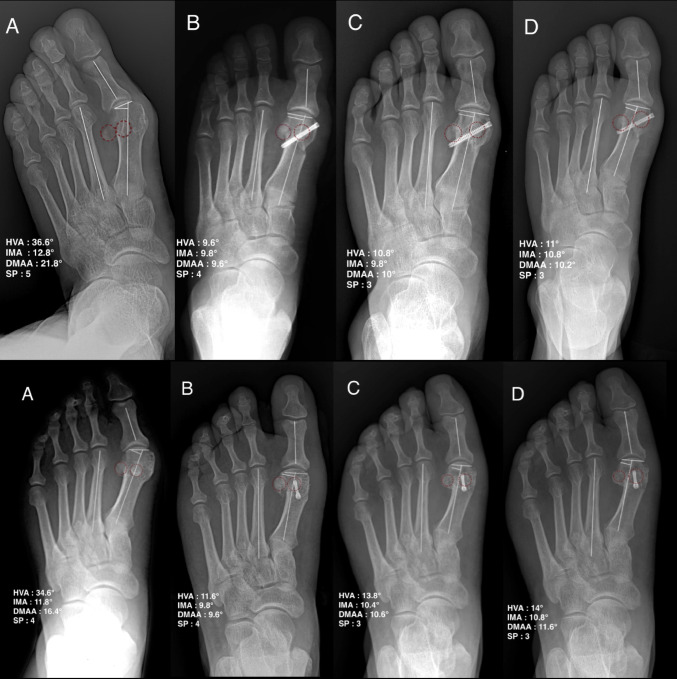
Baseline-matched weight-bearing anteroposterior (AP) radiographs (non-recurrent): Modified (upper) and Chevron (lower). A preoperative, B 6-8 weeks, C 1 year; D final. HVA/IMA/DMAA/sesamoid position annotated; red circles indicate the sesamoid complex.
